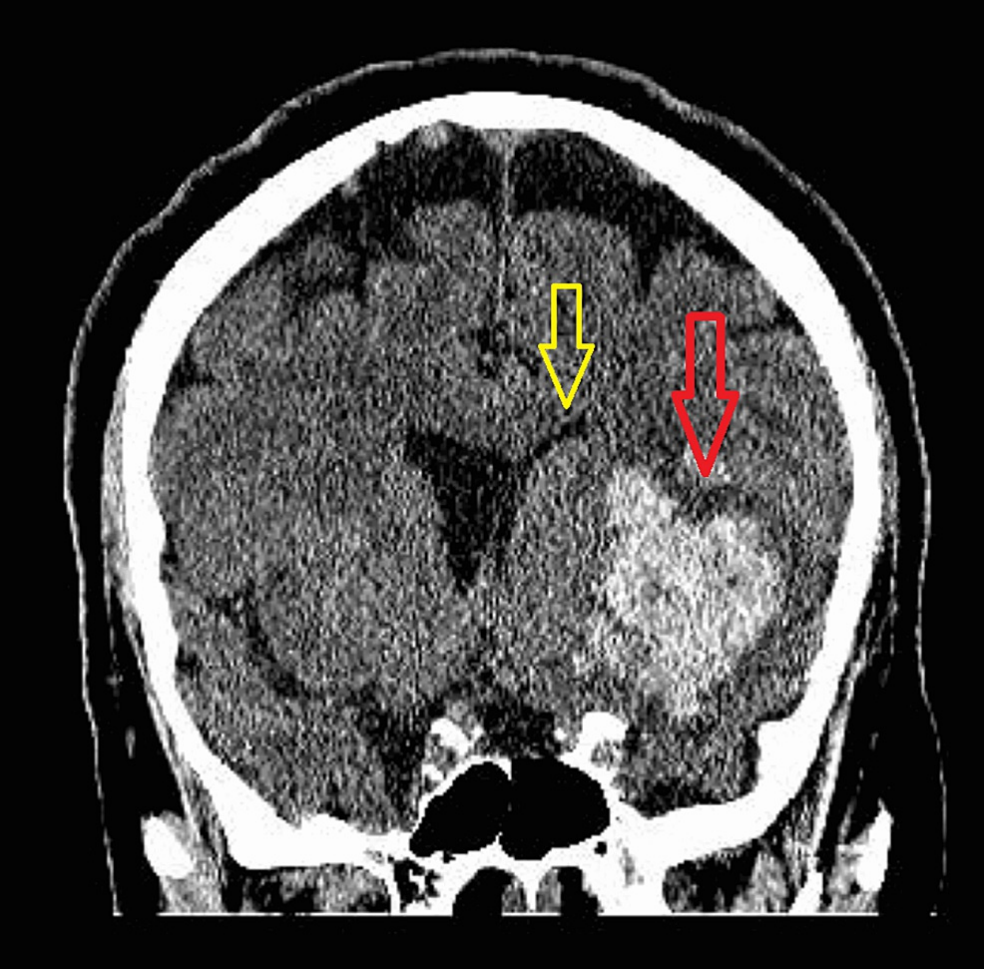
Computed tomography of the head: coronal view showing the effacement of the left lateral ventricle frontal horn and 5-mm left to right midline shift (yellow arrow) secondary to intraparenchymal hemorrhage (red arrow)
